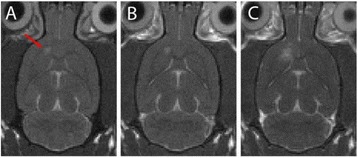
a T1weighted images before contrast administration. The red arrow indicates the tumor. b No difference in enhancement of the tumor is observed after contrast administration (ΔSI = 0.4 %). c After focused ultrasound-mediated Blood-Brain Barrier disruption, the tumor enhances after contrast administration (ΔSI = 30.1 %)
